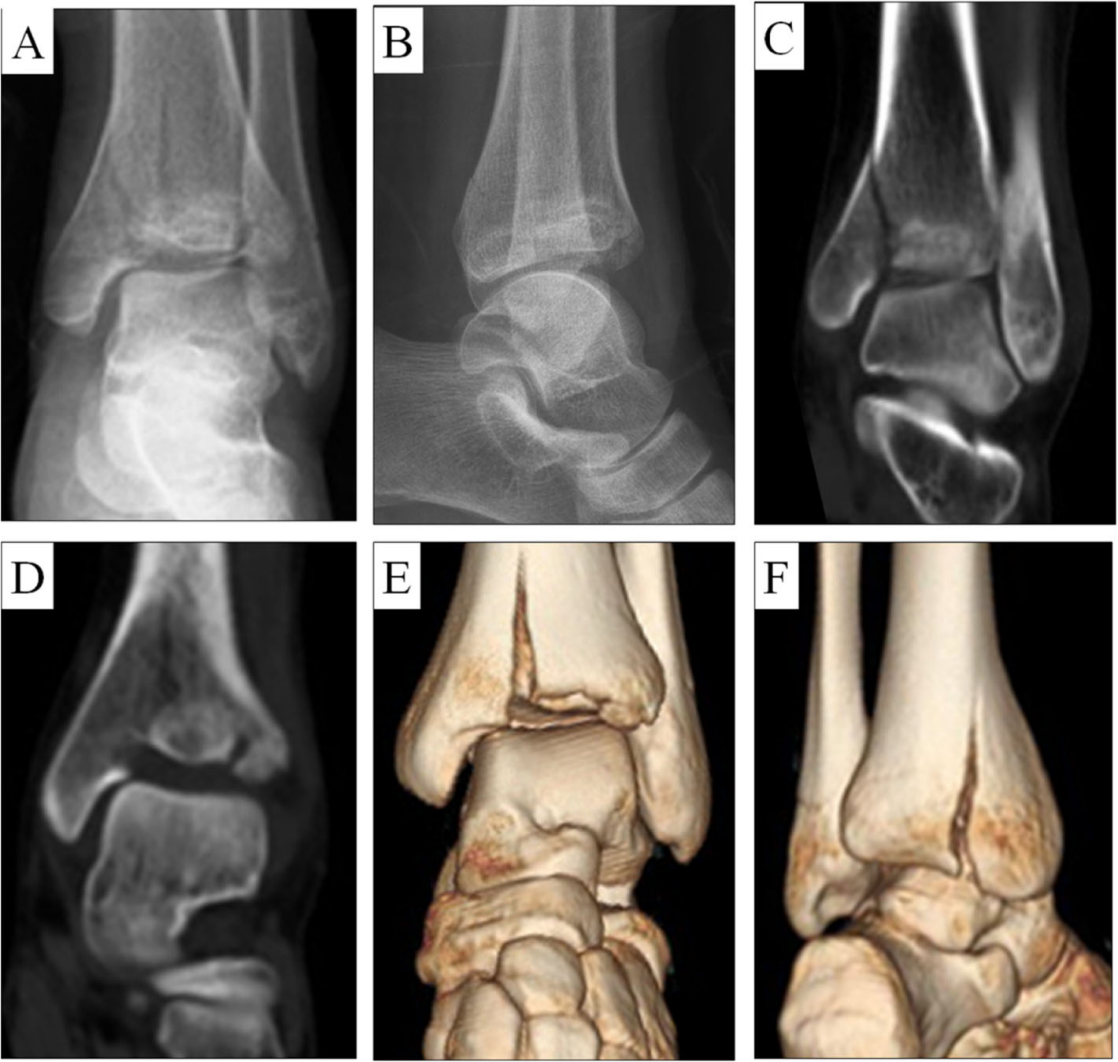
A female patient diagnosed with a 43-B2 Pilon fracture. a-b Preoperative X-rays and (c-f) three-dimensional CT showed the splitting and depression of the distal tibial articular surface. The Pilon fracture was combined with medial and lateral malleolus fractures
